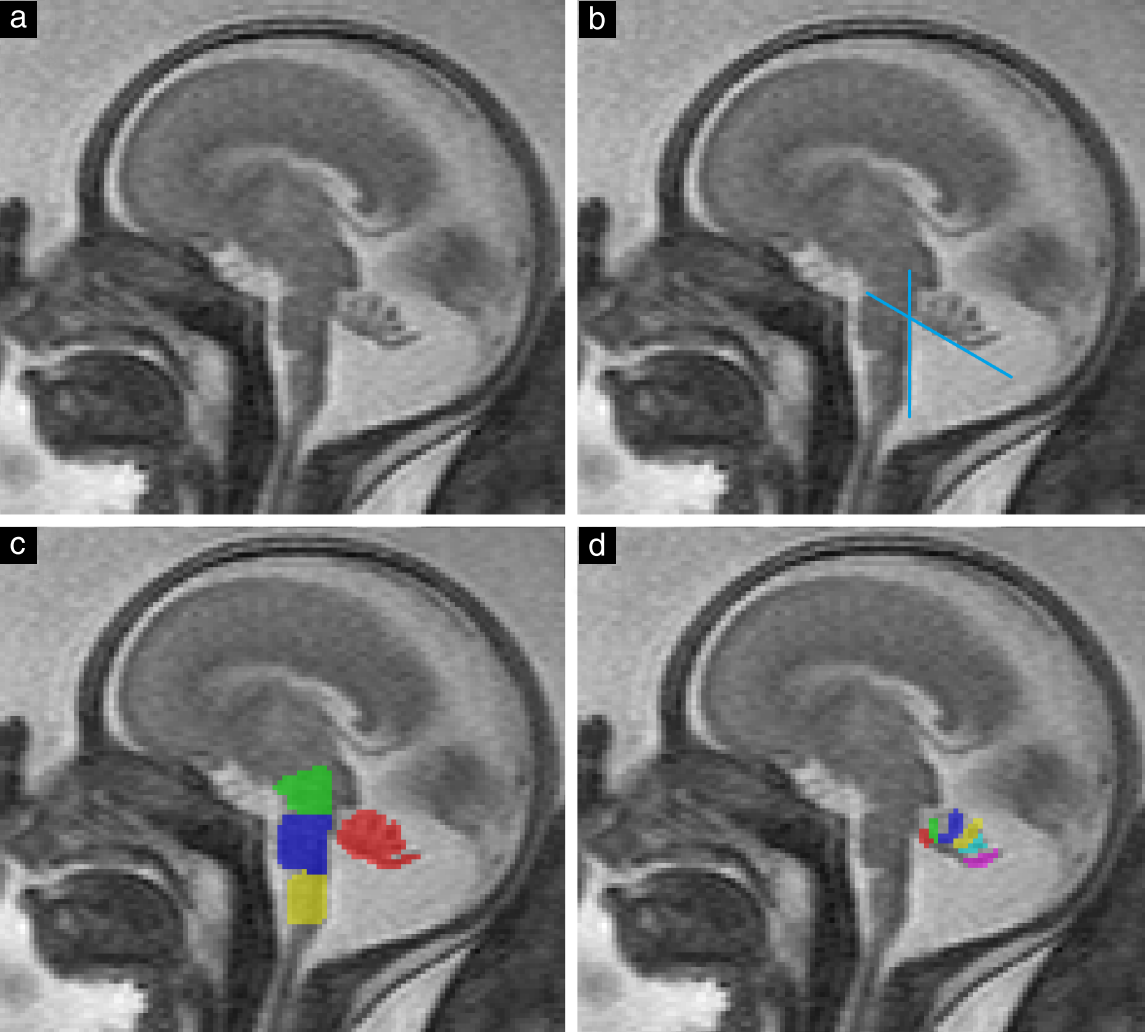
(a) Midsagittal T2‐weighted magnetic resonance image of fetal brain at 23 + 4 weeks' gestation. (b) Brainstem–vermis angle (here, 60°) measured between dorsal border of brainstem at level of pons and ventral tangent of uprotated cerebellar vermis. (c) Areas of vermis (red), mesencephalon (green), pons (blue) and medulla oblongata (yellow) were segmented. (d) Number of differentiable lobules (six in this case) was assessed.
